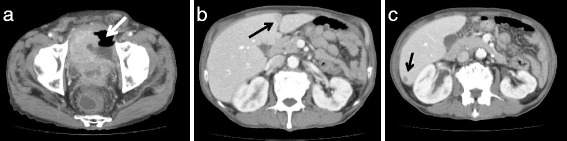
The findings of contrast computed tomography (CT). The huge tumor was occupying in minor pelvic space and invading to the bladder and small intestine. *White arrow* indicates the urinary bladder with tumor invasion and air-fluid level. a
